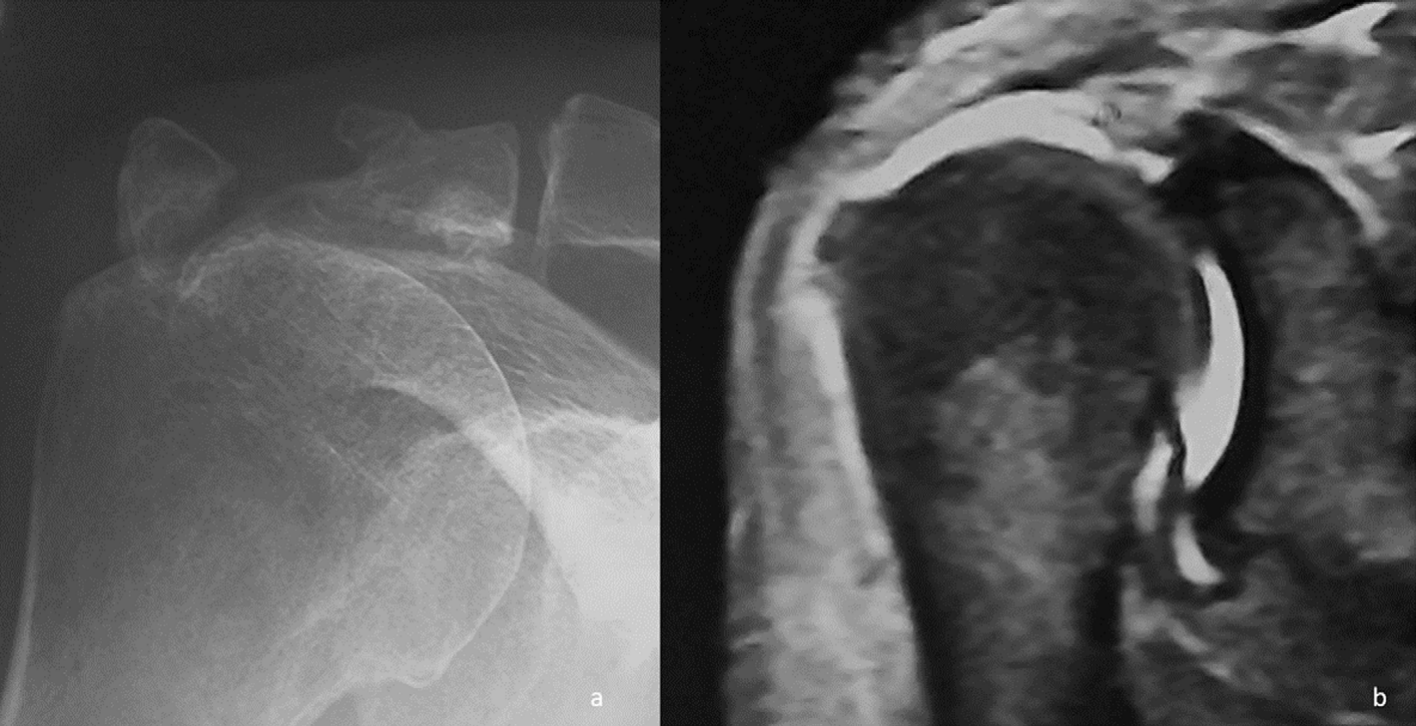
37-year-old patient with a history of right shoulder trauma of 2 months of evolution. He was treated with three local injections of platelet-rich plasma. a) X-rays showed a displaced acromion fracture and magnetic resonance showed a massive rotator cuff tear. Clearly, the initial indication was surgical.
